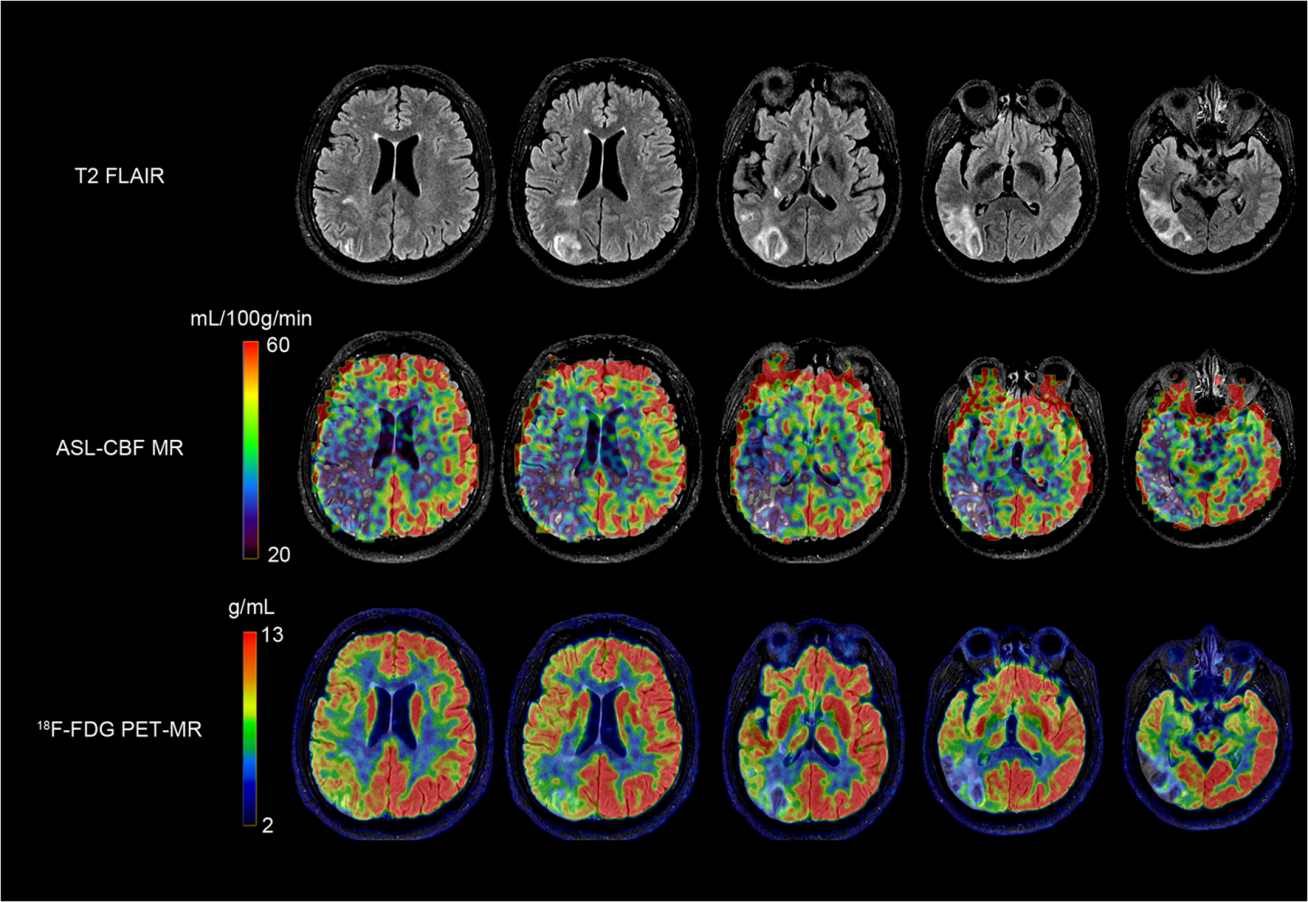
A 43-year-old man with symptomatic occlusion of the right ICA. T2 FLAIR images acquired at baseline showed infarction in the right occipital and temporal lobe. Both ASL-CBF and ^18^F-FDG PET showed that CBF and ^18^F-FDG uptake (right) were reduced relative to finding in the contralateral hemisphere, with CBF AI and SUVR AI values of 37.55% and 30.73%, respectively
